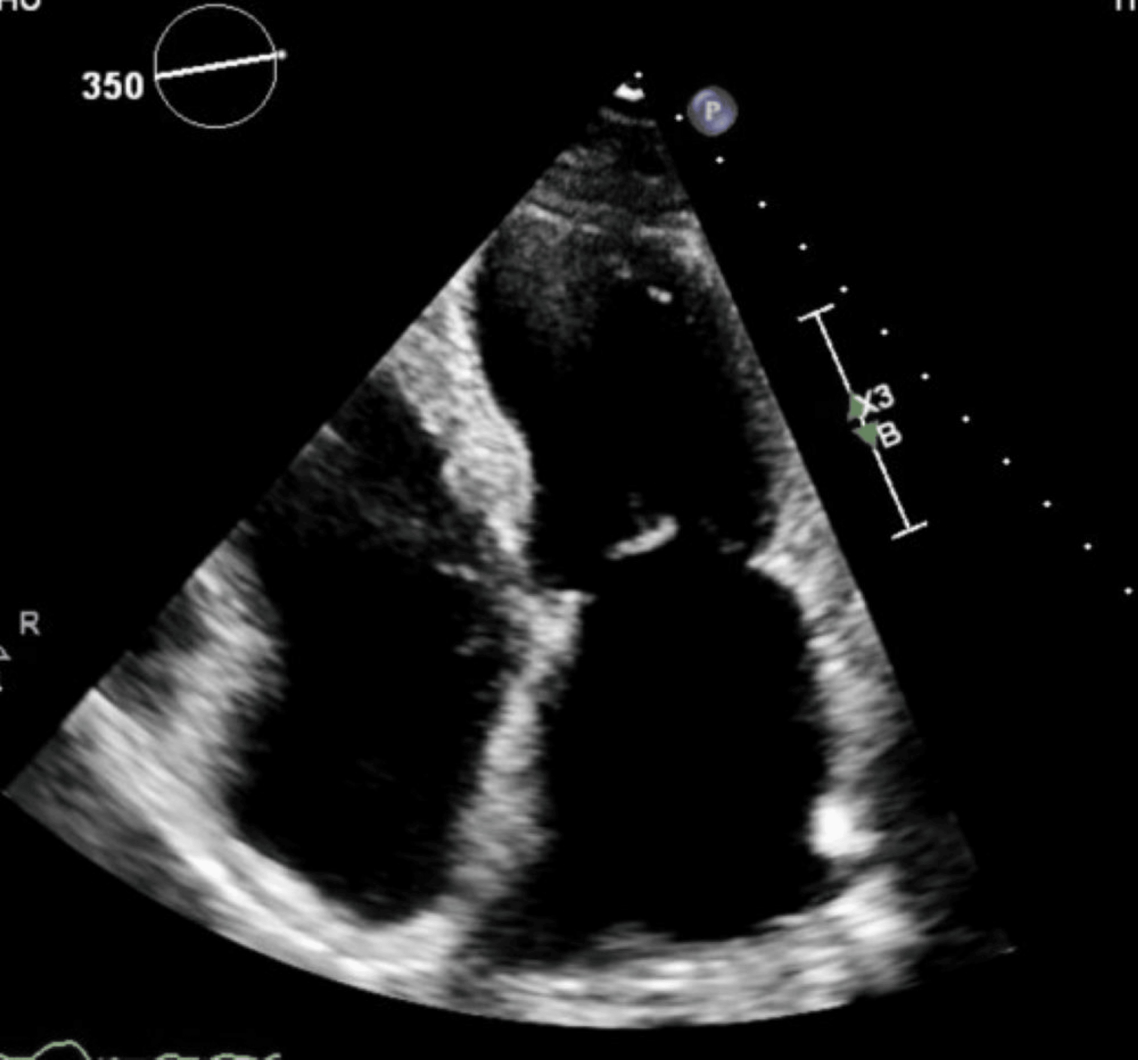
Transthoracic echocardiogram image
